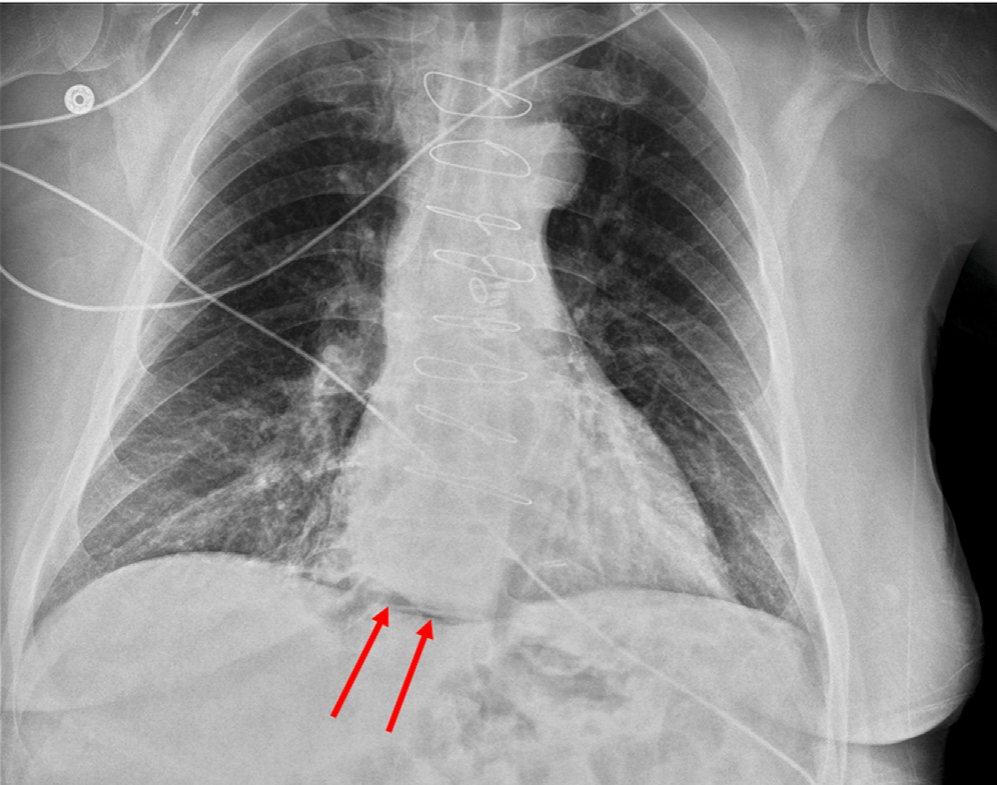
Chest radiograph with lucency in inferior mediastinum (*red arrows*).
